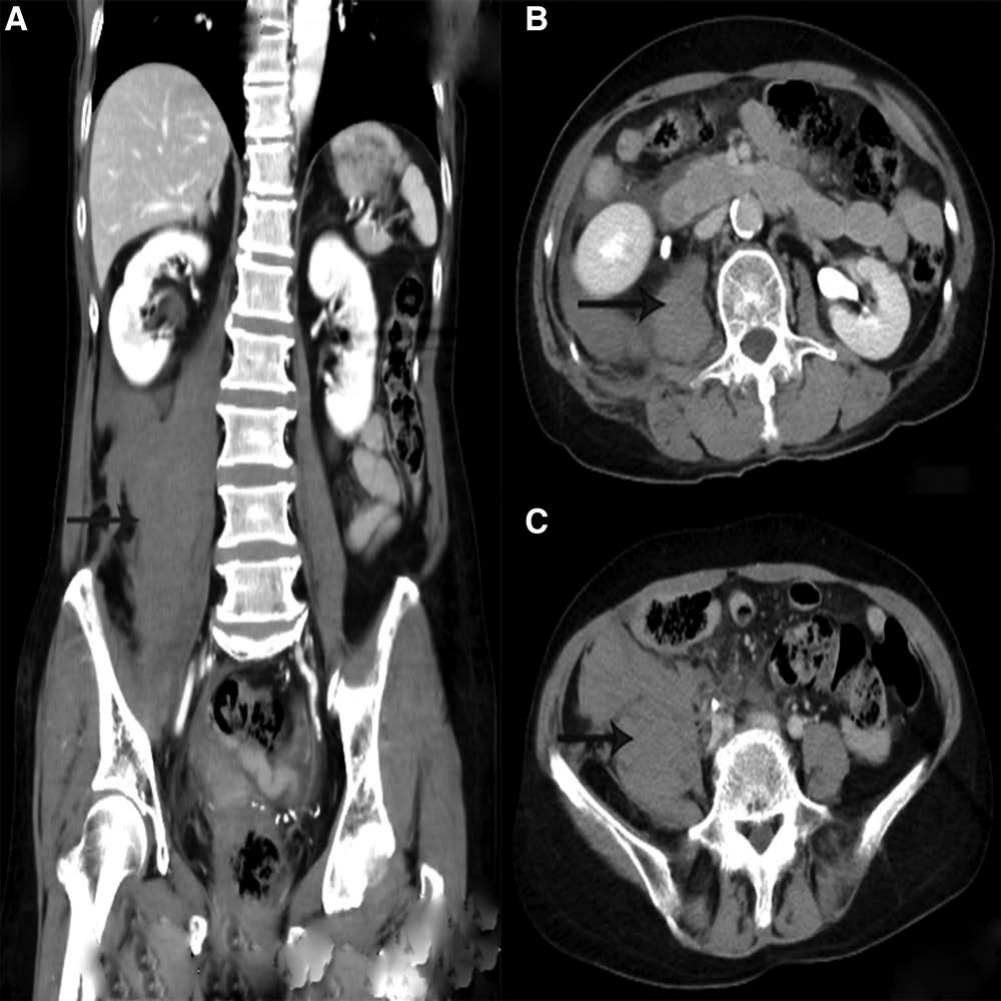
Contrast-enhanced CT image of the abdomen obtained about 9 h after the treatment of small needle scalpel. (a) Coronal view; (b) horizontal view at the L2 level; (c) horizontal view at the L5 level. CT = computed tomography.
